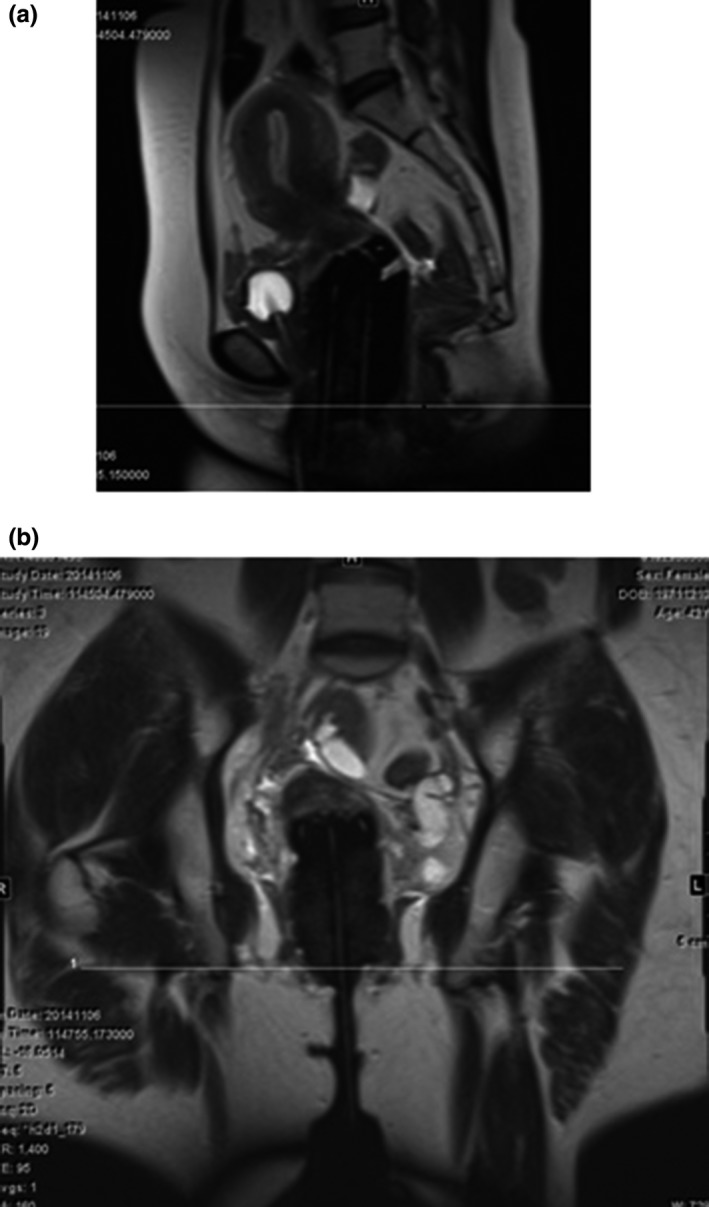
MRI sagittal view (a) and coronal view (b). Make sure to have sufficient margin (5 cm) above the uterus. MRI, magnetic resonance imaging.
